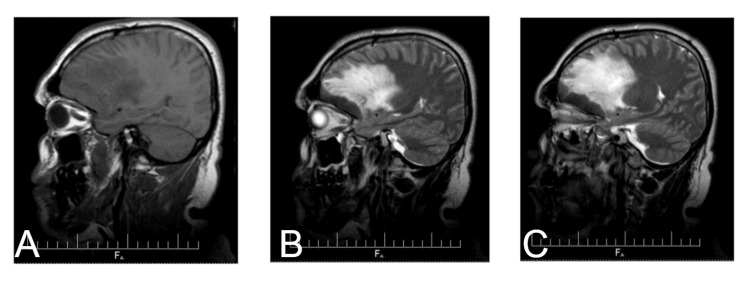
Sagittal MRI images of the brain. (A) An MRI T1-weighted image sagittal view shows a hypo- to iso-intense lesion associated with edema. The lesion appears well-defined, without irregular borders, which is consistent with meningiomas. (B) A T2-weighted image shows lesion at the anterior skull base, involving the frontal region. (C) Further delineation of the lesion shows increased hyperintensity (brain edema) in the T2 and involvement of the sphenoid wings and optic chiasm.
